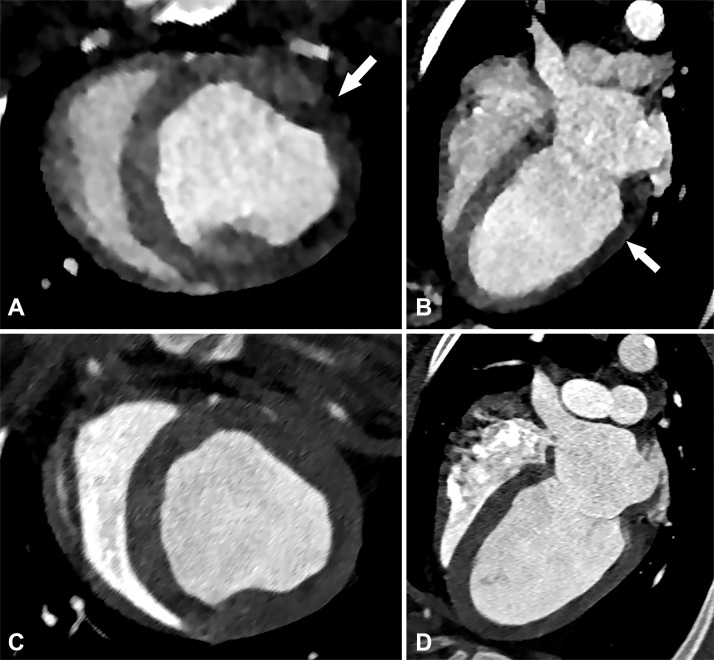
Perfusion defect in a 54-year-old man with chest pain. Short-axis, *A,* and four-chamber, *B,* CT images from a dynamic CT stress perfusion examination show a large transmural perfusion defect within the lateral wall (arrows), corresponding to a hemodynamically significant left circumflex lesion. Short-axis, *C,* and four-chamber, *D,* rest perfusion CT images are normal.
